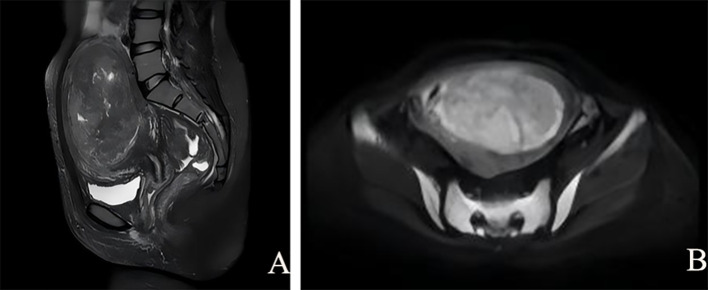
Pelvic magnetic resonance imaging (MRI). (A) Sagittal T2-weighted imaging (T2WI) demonstrates a large mass lesion within the uterine myometrium. The lesion shows intermediate-to-low signal intensity on T2WI, with heterogeneous areas of slightly hyperintense and markedly hyperintense signal. (B) Diffusion-weighted imaging (DWI) shows mildly hyperintense signal within the lesion.
